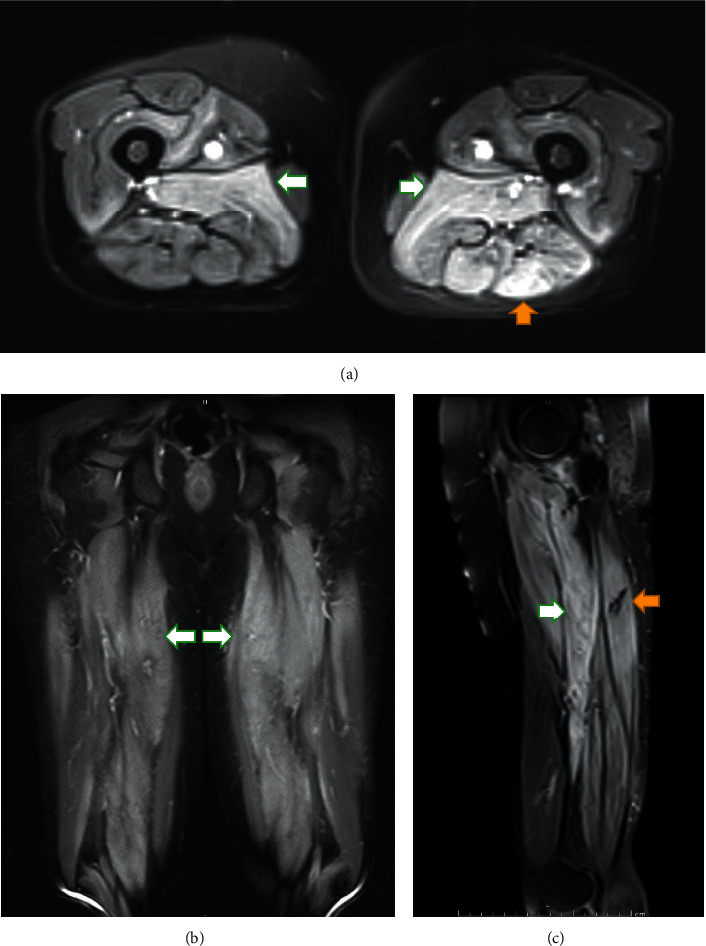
MRI of both thighs ((a) T2 tse fat sat axial, (b) T2-tirm-fs coronal, and (c) T2 tse-fs sagittal left thigh) showing a considerable degree of slightly heterogenous relative hyperintensity of the adductor muscles of both thighs (white arrows) as well as the hamstring muscles of the left thigh (yellow arrows) with no gross increased volume of the muscles appearance. These findings raise the possibility of partial atrophy and intrasubstance edema possibly due to the effects of chronic myositis/myopathy with secondary early muscle atrophic changes.
